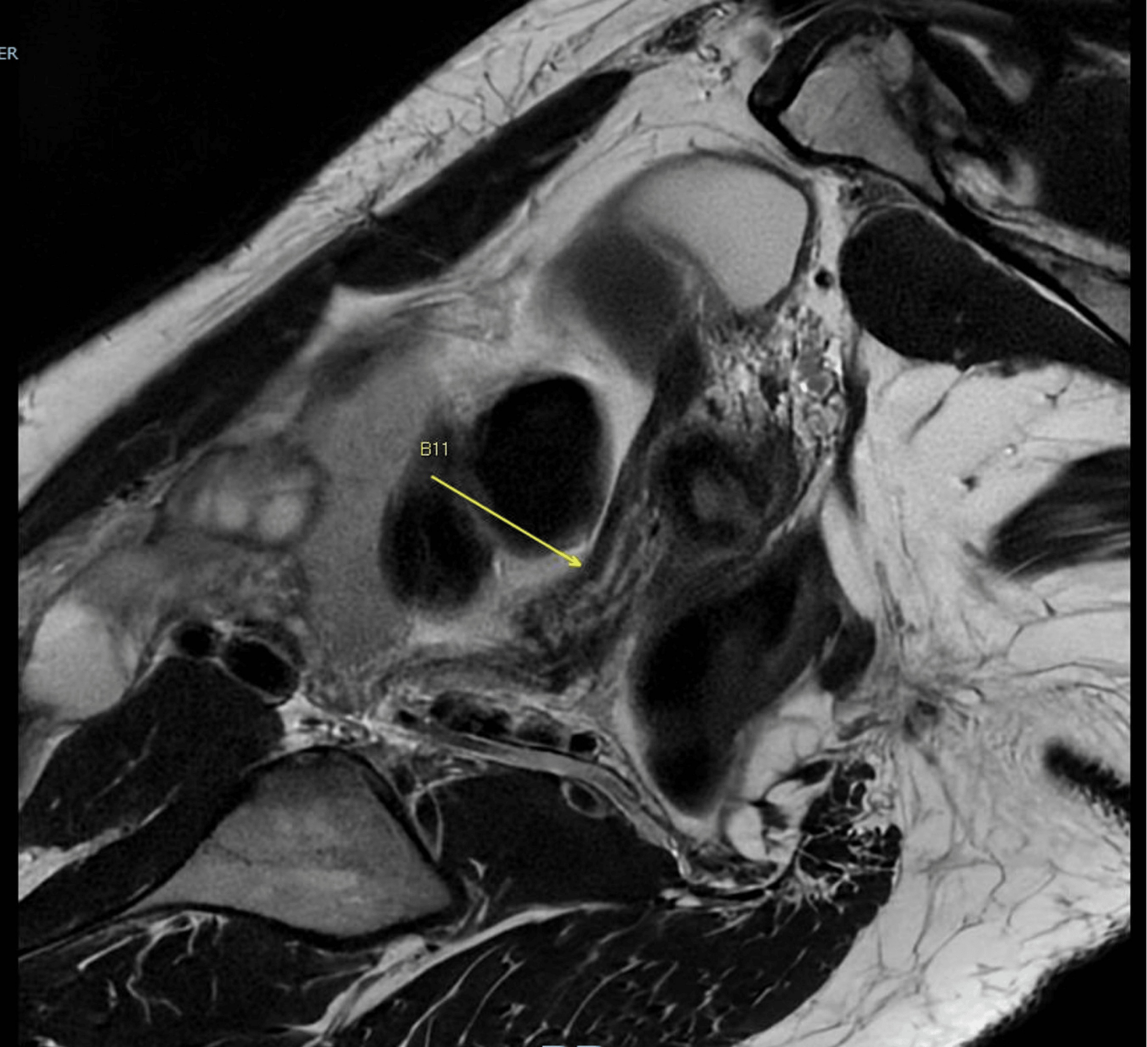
MRI image showing the right uterine horn communicating with a severely narrow right cervix MRI: magnetic resonance imaging
